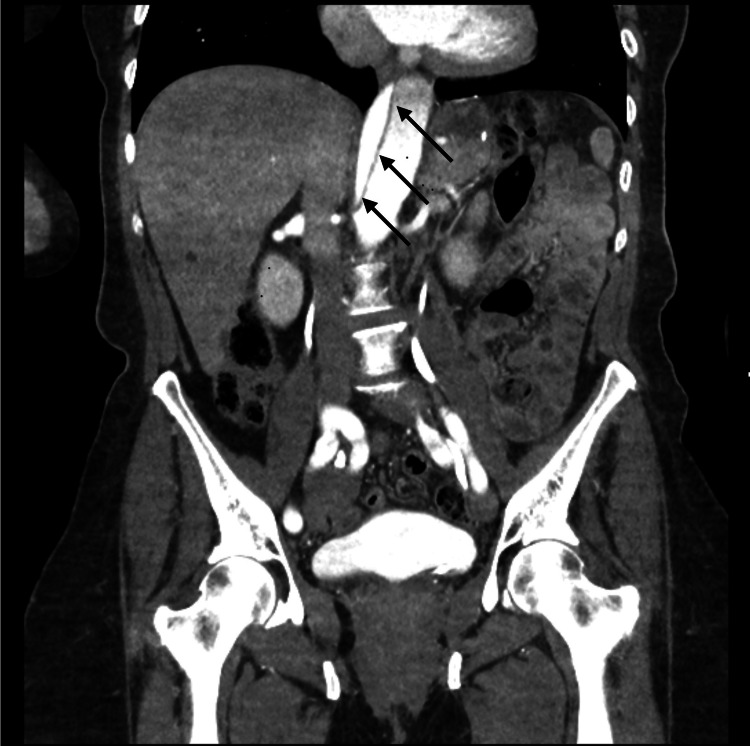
CTA of the abdomen and pelvis coronal image showing an alternative view of the repaired DeBakey type 1 dissection. Arrows indicate the false lumen of the dissection CTA: computed tomography angiography
